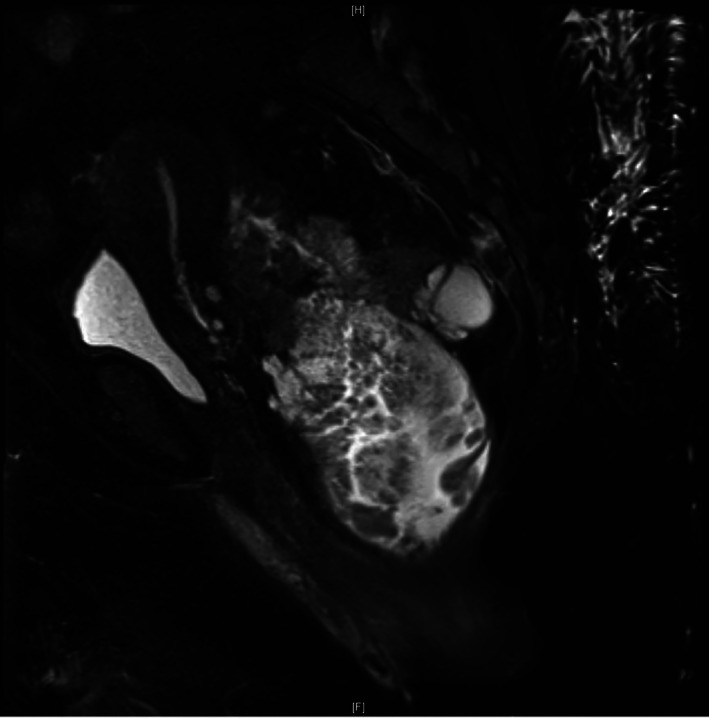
MRI pelvis demonstrated a circumferential, fungating, mucinous, 15.6 cm mass within 1 cm of the anal verge, extending from above the peritoneal reflection down to the lower rectum and upper anal canal. The tumor involved the puborectalis, and internal and external anal sphincters, and was noted to have a 50‐mm depth of invasion. Due to abutment of the posterior cervix, vaginal cuff, and bilateral levator muscles, the tumor was believed to invade adjacent organs and was staged as a T4b tumor. The MRI was also suspicious for local lymph node involvement; therefore, the patient was deemed to be clinical stage IIIC (T4b, N1, Mx)
